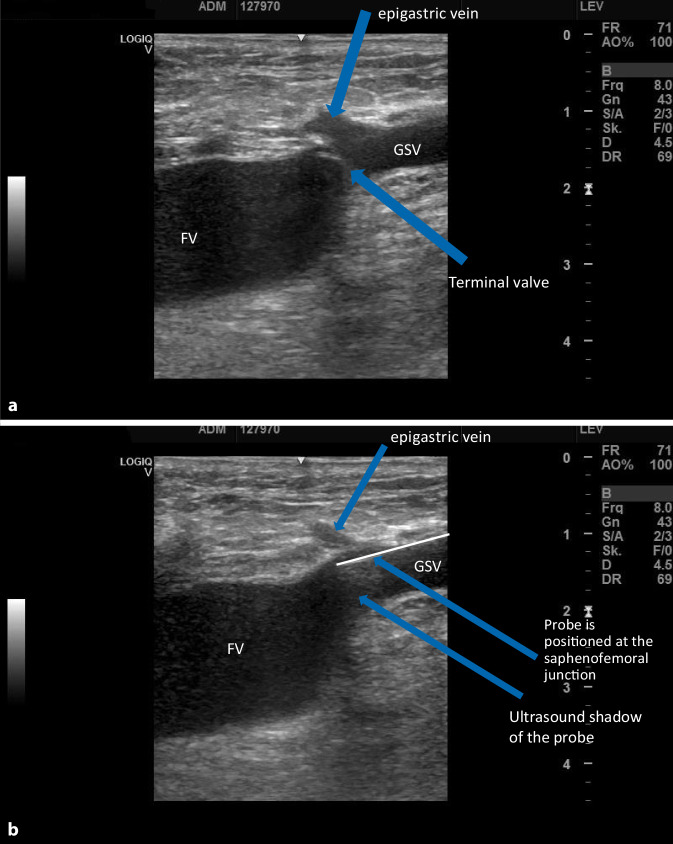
Duplex ultrasound: positioning the probe at the saphenofemoral junction. Endovenous crossectomy with evenly distributed occlusion of the long saphenous vein. a Before insertion of the probe. b Probe is in position. *FV* femoral vein, *GSV* great saphenous vein
